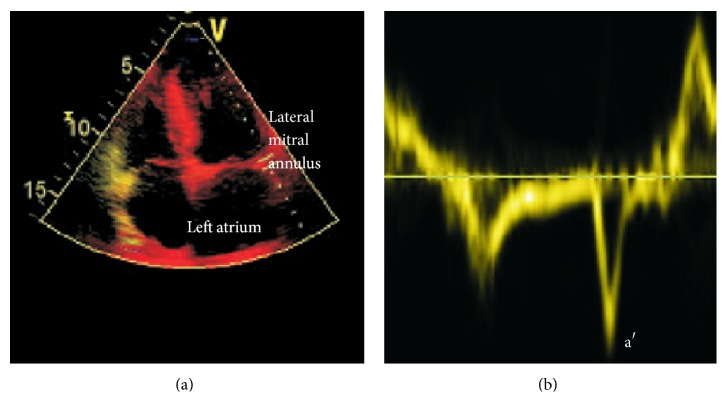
Measurement of left atrial velocity using tissue Doppler. The left image demonstrates the position of the probe at the lateral mitral annulus. The right image represents the velocities generated during diastole with the atrial component (termed a′) marked (unpublished).
